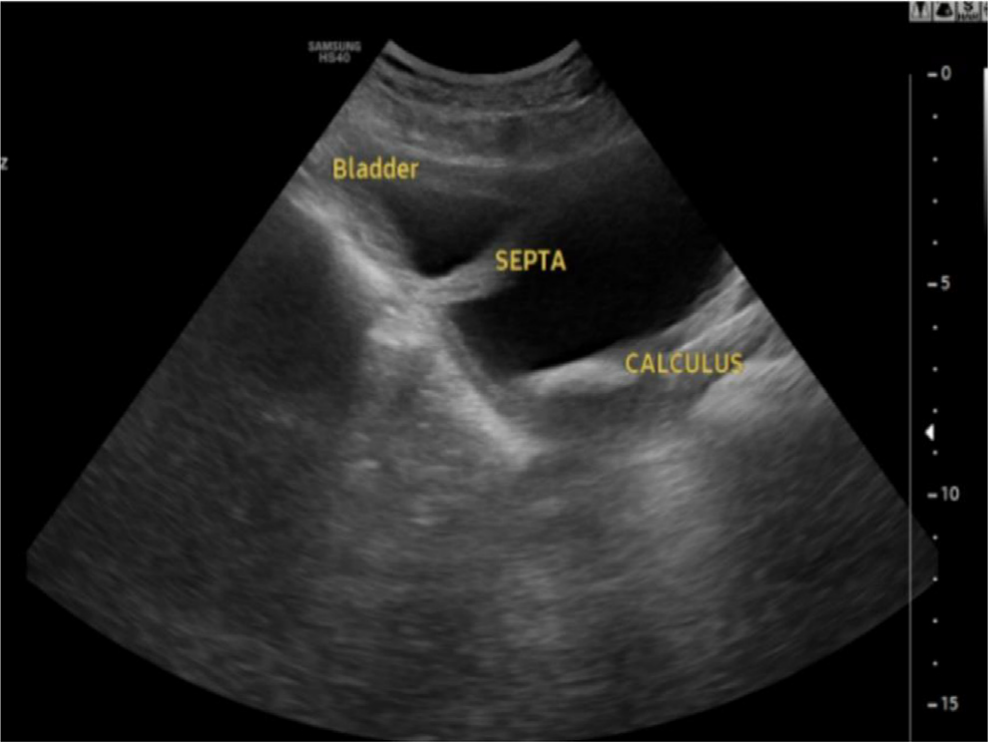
Abdominal ultrasound image (axial plane) showing thick hyperechoic septum dividing the bladder into 2 cavities with multiple free floating internal echoes and a calculus in left compartment of the bladder.
